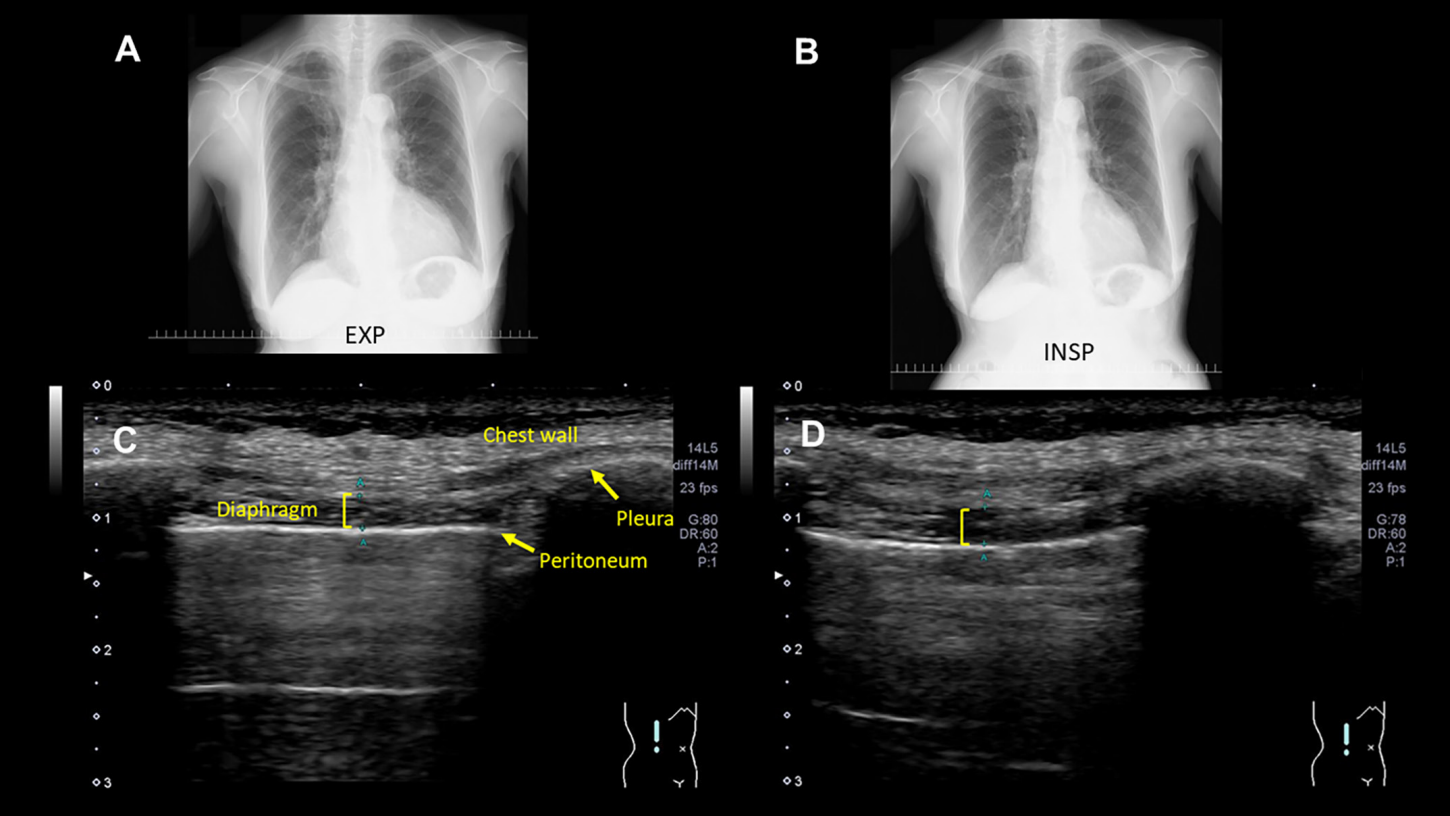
Chest radiographs during maximum expiration (A) and inspiration (B) and diaphragmatic ultrasound assessment (C). Chest radiographs show little change in the height of the diaphragm during maximum expiration (A) and inspiration (B). Ultrasound evaluation demonstrating minimal diaphragmatic thickening during maximum inspiration. Left panel (C) shows the thickness was 2.5 mm at end‐expiration (TDE) and right panel (D) shows it was 2.8 mm at end‐inspiration (TDI). This represents a thickening fraction (TDF) of 12% ((TDI − TDE)/TDE × 100), well below the normal threshold of 20%, indicating diaphragmatic dysfunction.
